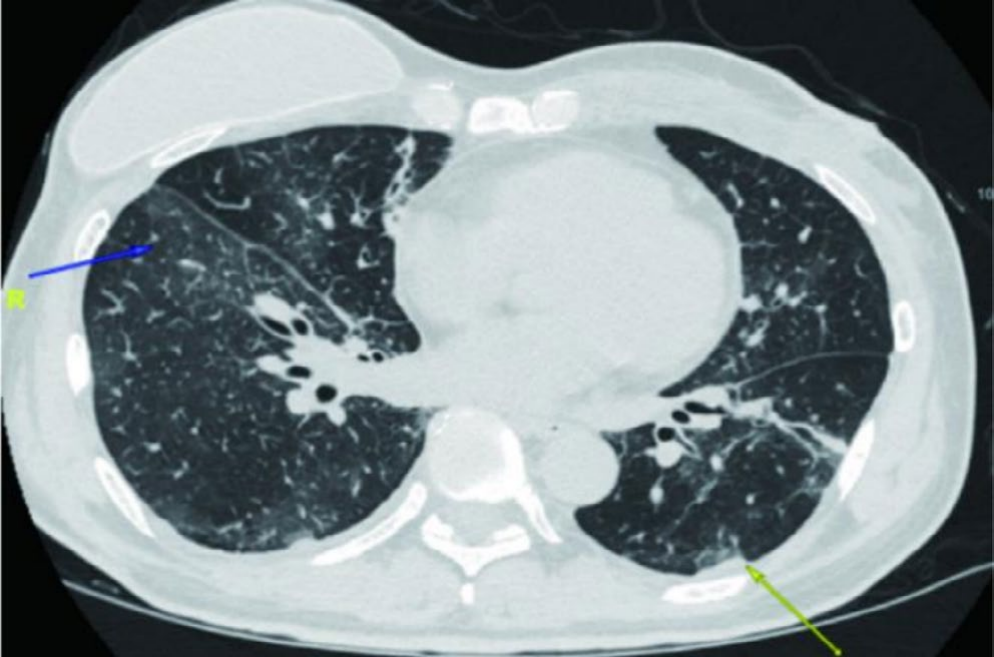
Bilateral interstitial inflammation with subpleural exudation and thickening on chest CT.
